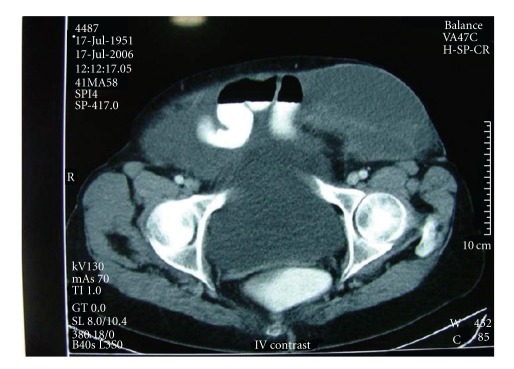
Magnetic resonance imaging of the case (IV contrast, T1). A large incisional hernia containing bowel loops and a great amount of fluid.
